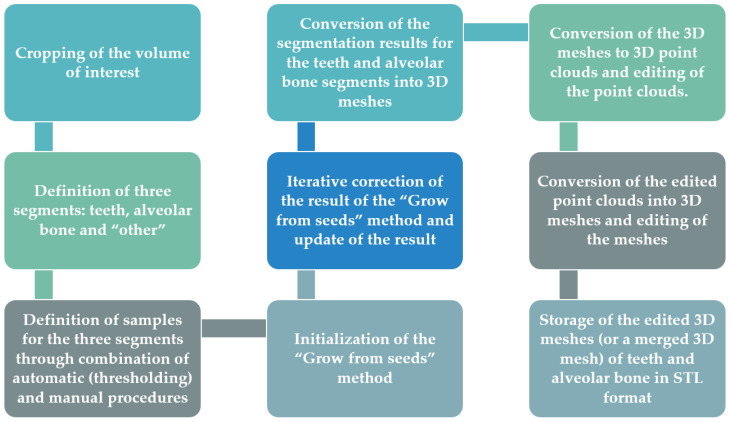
Proposed methodology for 3D modeling of the hard tissues of the oral system using dental CBCT data.
